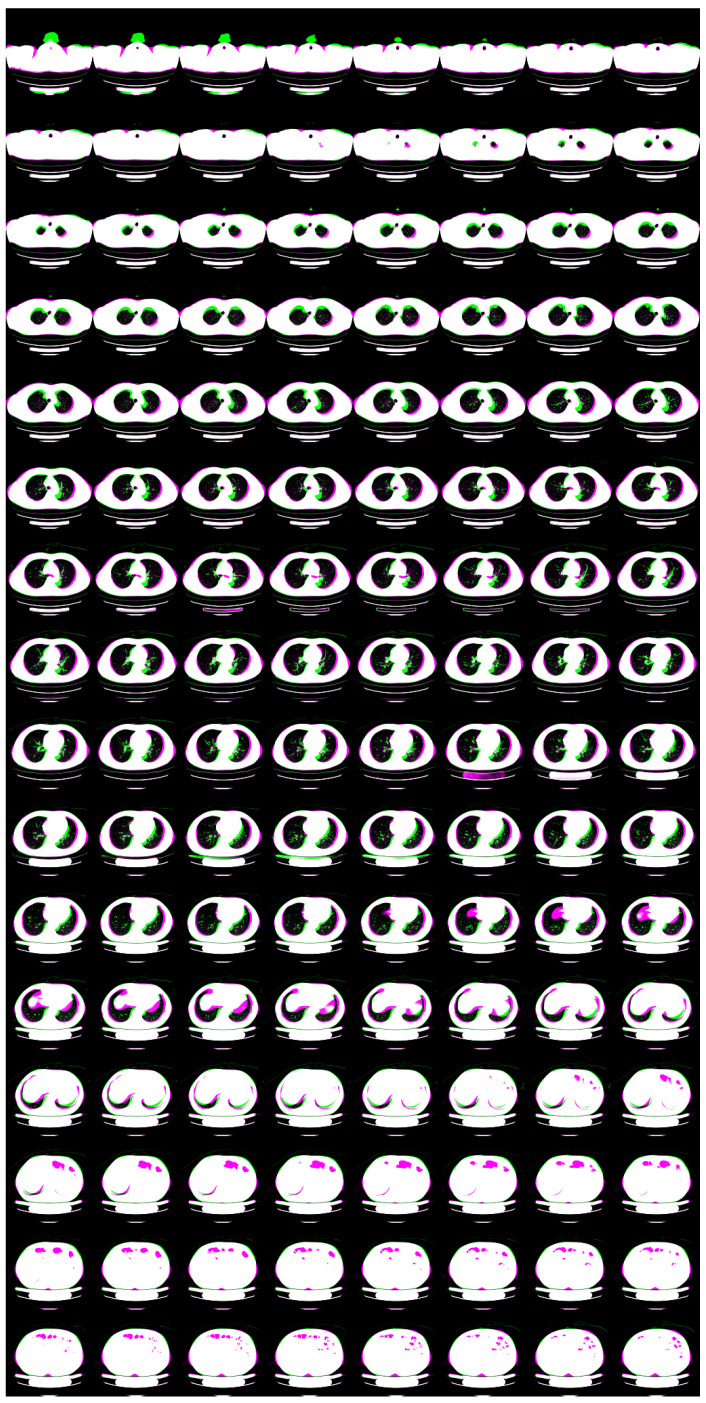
Superposition of the reconstructed 3D CT image shown in Figure 8 (magenta) and the ground-truth image (green).
